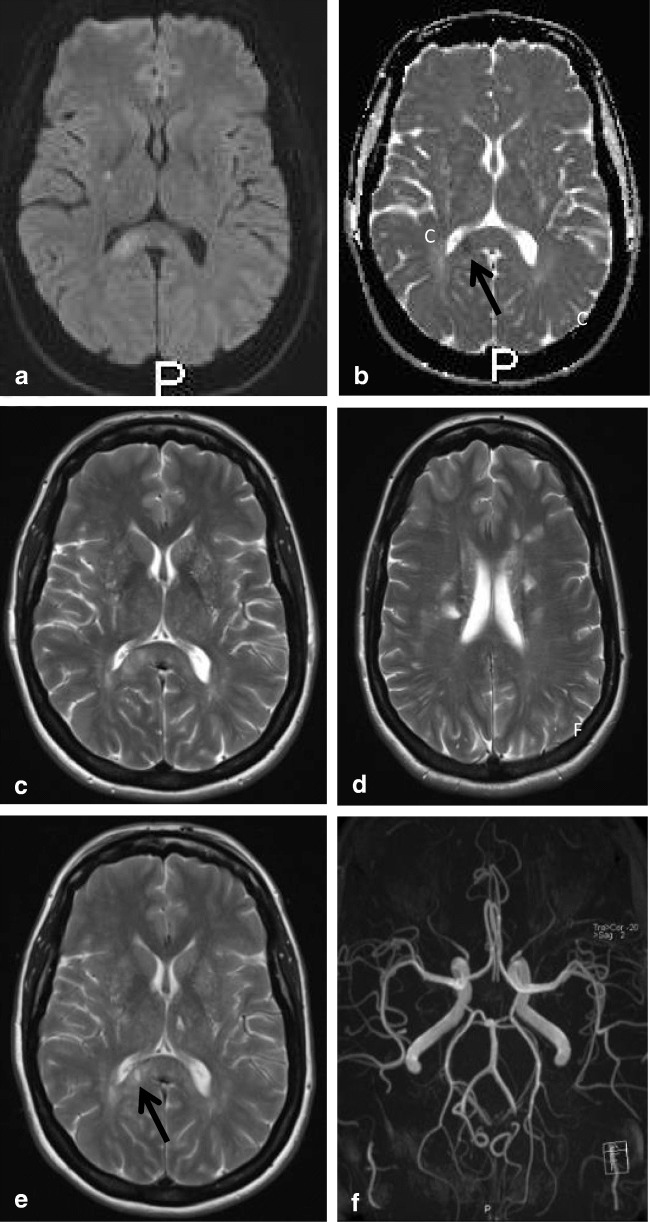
Forty-eight-year-old woman. Axial DWI (a) and ADC (b) show a small area of diffusion restriction in the right splenium (black arrow) with a larger area of edema on the T2 (c). In the corona radiata and frontal white matter, multiple lacunar infarcts are seen (d). Follow-up axial T2 (e) at the level of figure c shows residual focal loss of tissue in right splenium and a similar lesion in left thalamus (black arrow). 3D TOF MRA shows normal cerebral arteries (f)
